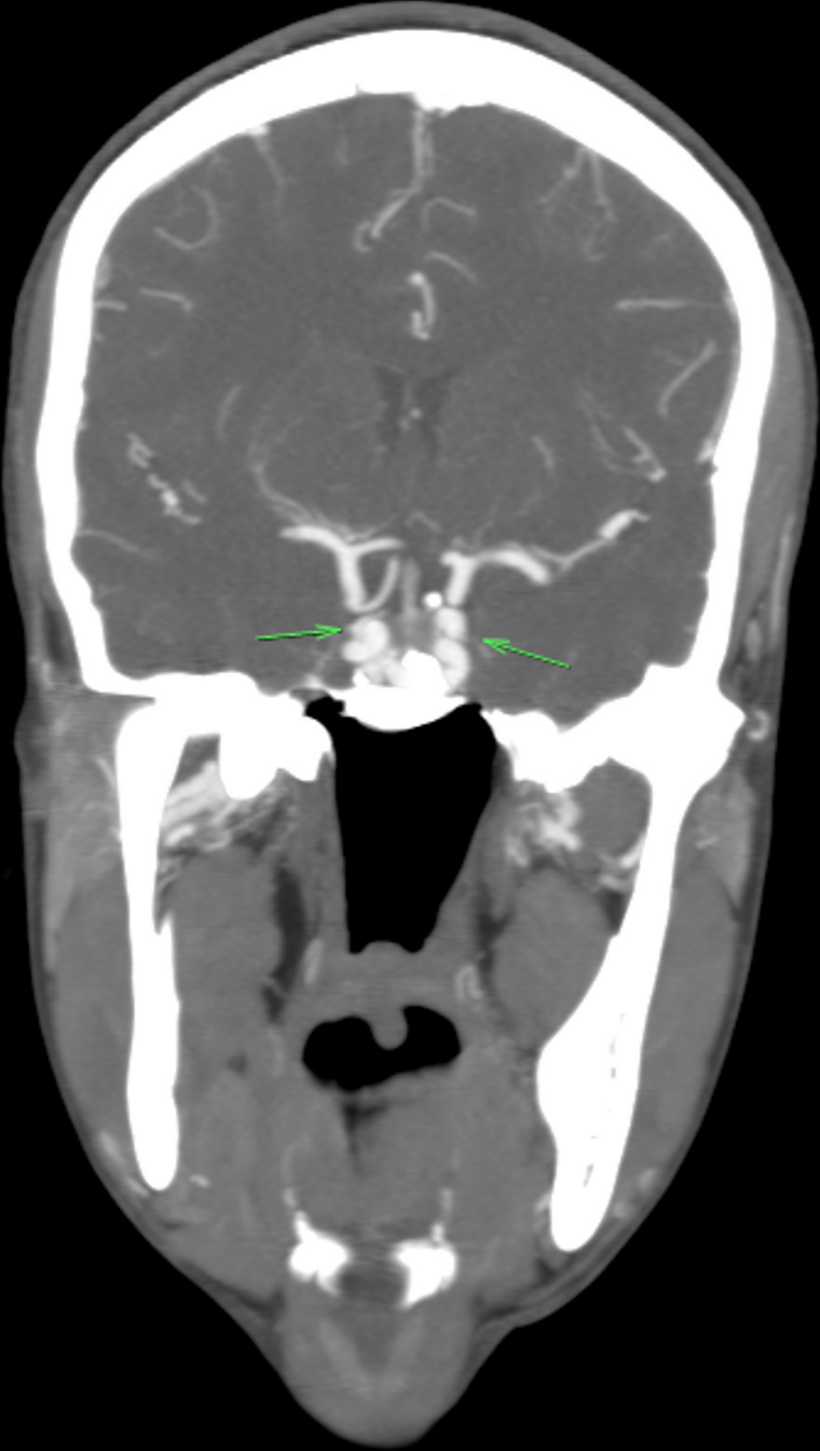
Coronal reformatted CTA image demonstrating a fused single ICA that runs through a solitary canal in the body of the clivus and bifurcates at dorsum sellae into right and left intra cranial very tortuous ICA’s as they continued intracranially to form respective arteries of the circle of Willis. CTA, CT angiography; ICA, internal carotidartery.
